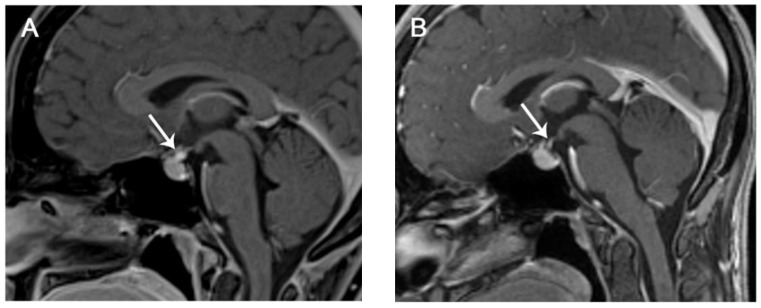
35-year-old with melanoma treated with Ipilimumab. After the third dose, she developed headache and nausea. Post-contrast T1-weighted MR sagittal images obtained at onset (A) and at the 6-month follow-up (B). Subtle thickening of the pituitary stalk is seen at the first MRI (arrow in (A)). Post-contrast T1-weighted MR image after discontinuation of Ipilimumab and application of steroid therapy showed normalization of the pituitary stalk (arrow in (B)), suggesting mild infundibular hypophysitis.
